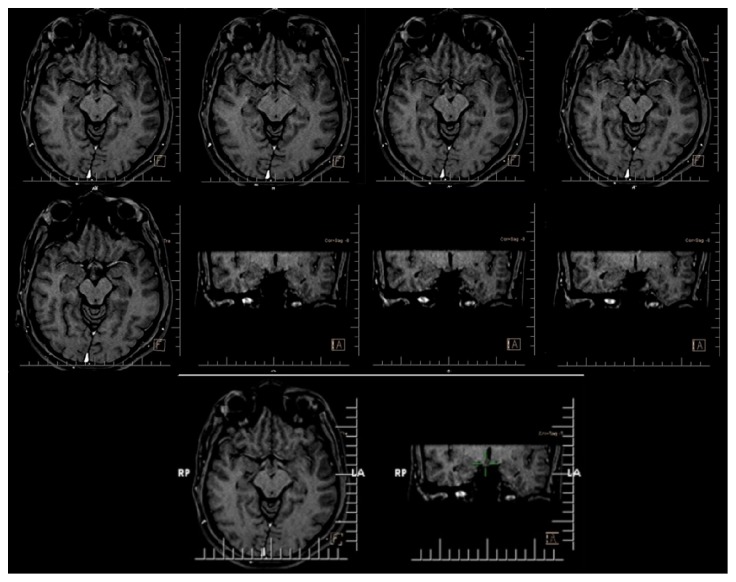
MRI of the patient revealing hypothalamic hamartoma.
